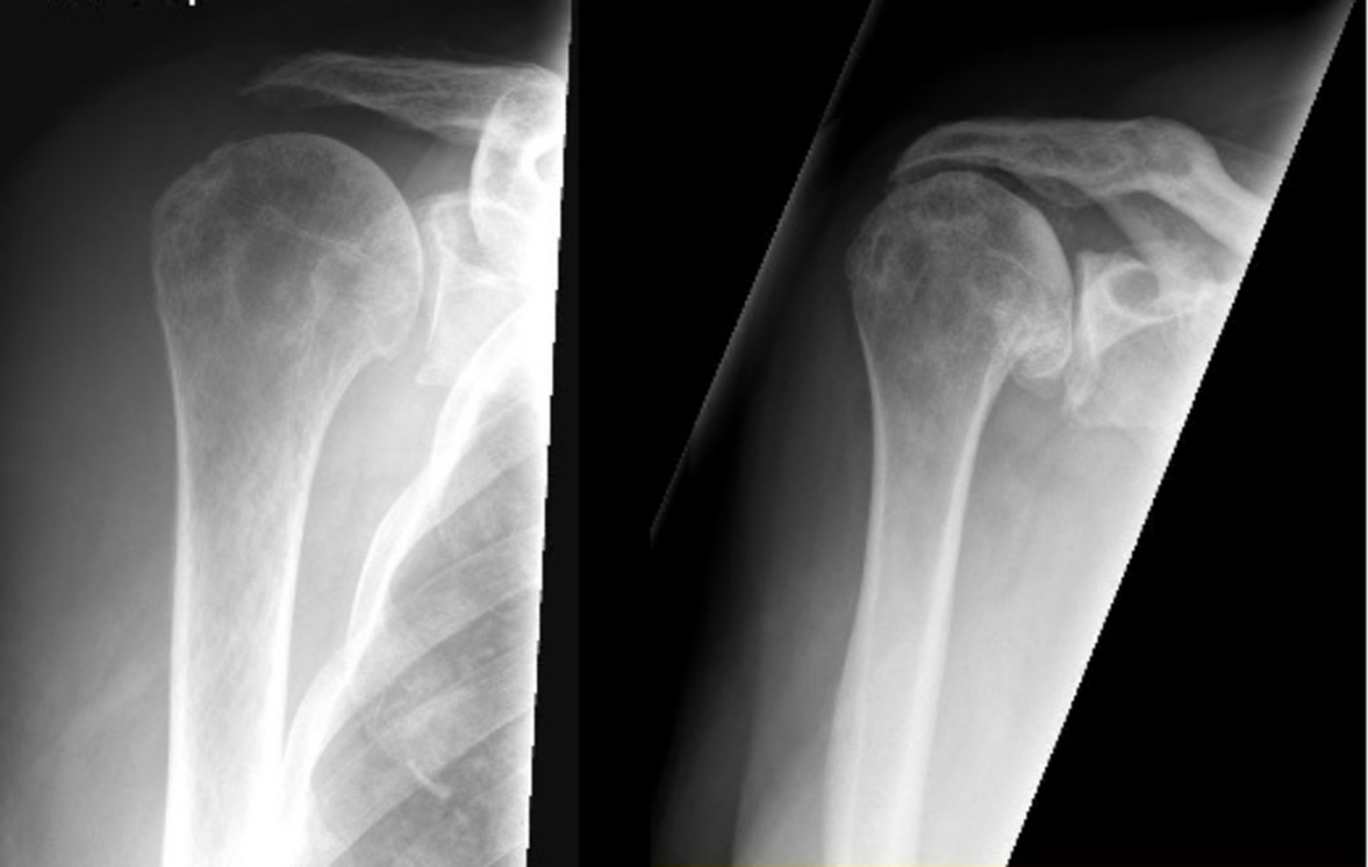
Preoperative (left) radiograph of a 79-year-old female patient with a Hamada grade 2; postoperative (right) radiograph showing a progression of the Hamada grade to 4(B) after 10 years.
